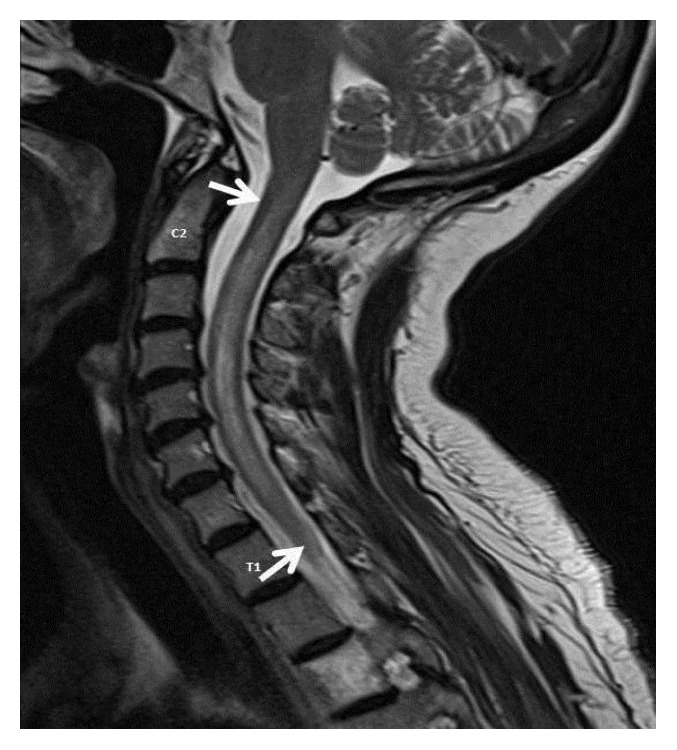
MRI of spine showing intramedullary demyelination in the spinal cord, evident as enhanced T2 signal, with peripheral contrast enhancement, extending from C2 to T1 vertebral body (arrows).
